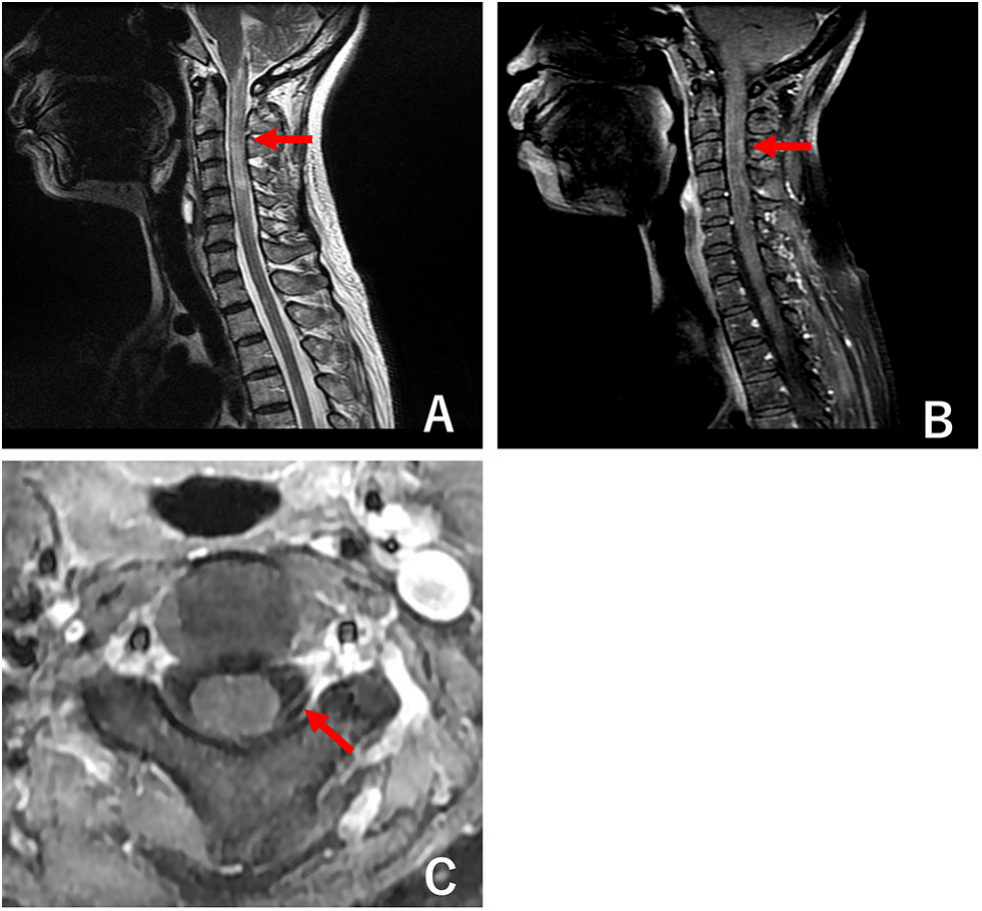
Magnetic resonance imaging (MRI) of cervical to thoracic spinal cord. (A) Sagittal T2 MRI showing longitudinally extensive transverse myelitis (arrow) from the lower part of the medulla oblongata to C5 with marked edema. (B) Gadolinium contrast MRI of cervical to thoracic spinal cord showing mild enhancement (arrow), which represents the active lesions of myelitis. (C) Axial gadolinium contrast MRI showing active radiculopathy of the left side (arrow).
